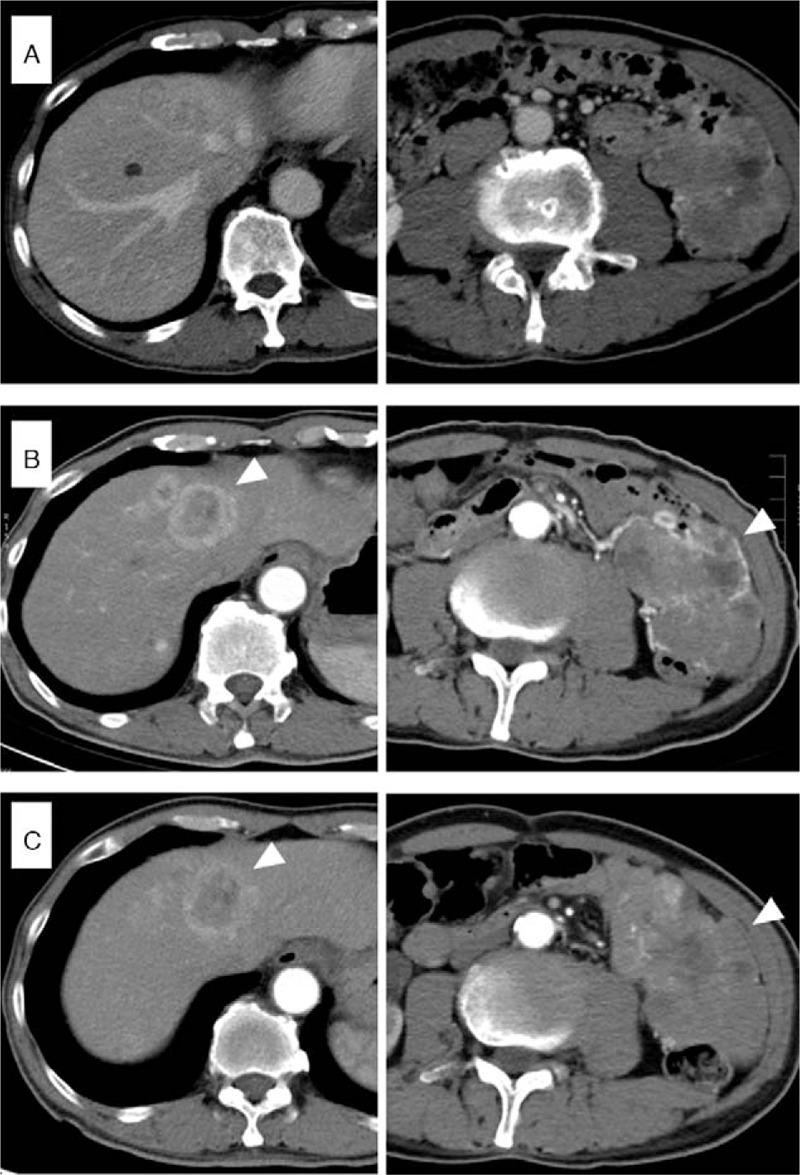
Abdominal computed tomographic images showing liver and peritoneal dissemination. The images show the tumors at the beginning of the treatment (A), following first-line treatment combining bevacizumab, Irinotecan, and S-1 (B), and after second-line treatment of bevacizumab, oxaliplatin, and HDFL (high-dose 24-hour infusion of 5-fluorouracil and leucovorin regimen) (C). Arrowheads indicate tumors with an increase in size.
